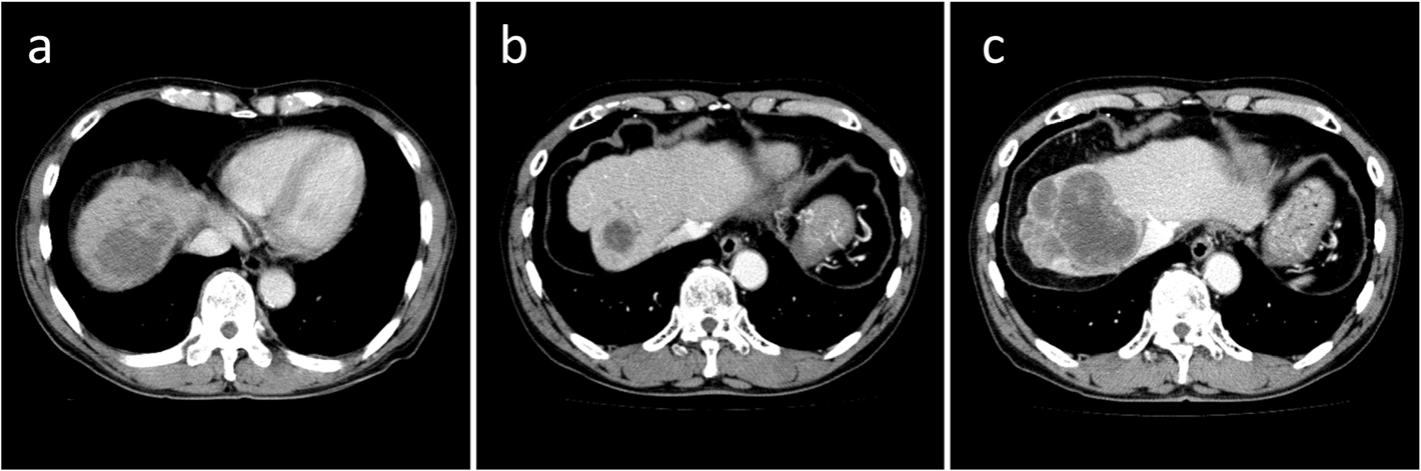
Enhanced CT images after introducing HAIC. Remarkable regression of intrahepatic lesions, thrombosis in the right portal vein and inferior vena cava is seen after one HAIC cycle (a). Tumor remained shrunk without treatment for 16 months after interrupting HAIC (b). Tumor progression occurred 18 months after the HAIC interruption (c)
